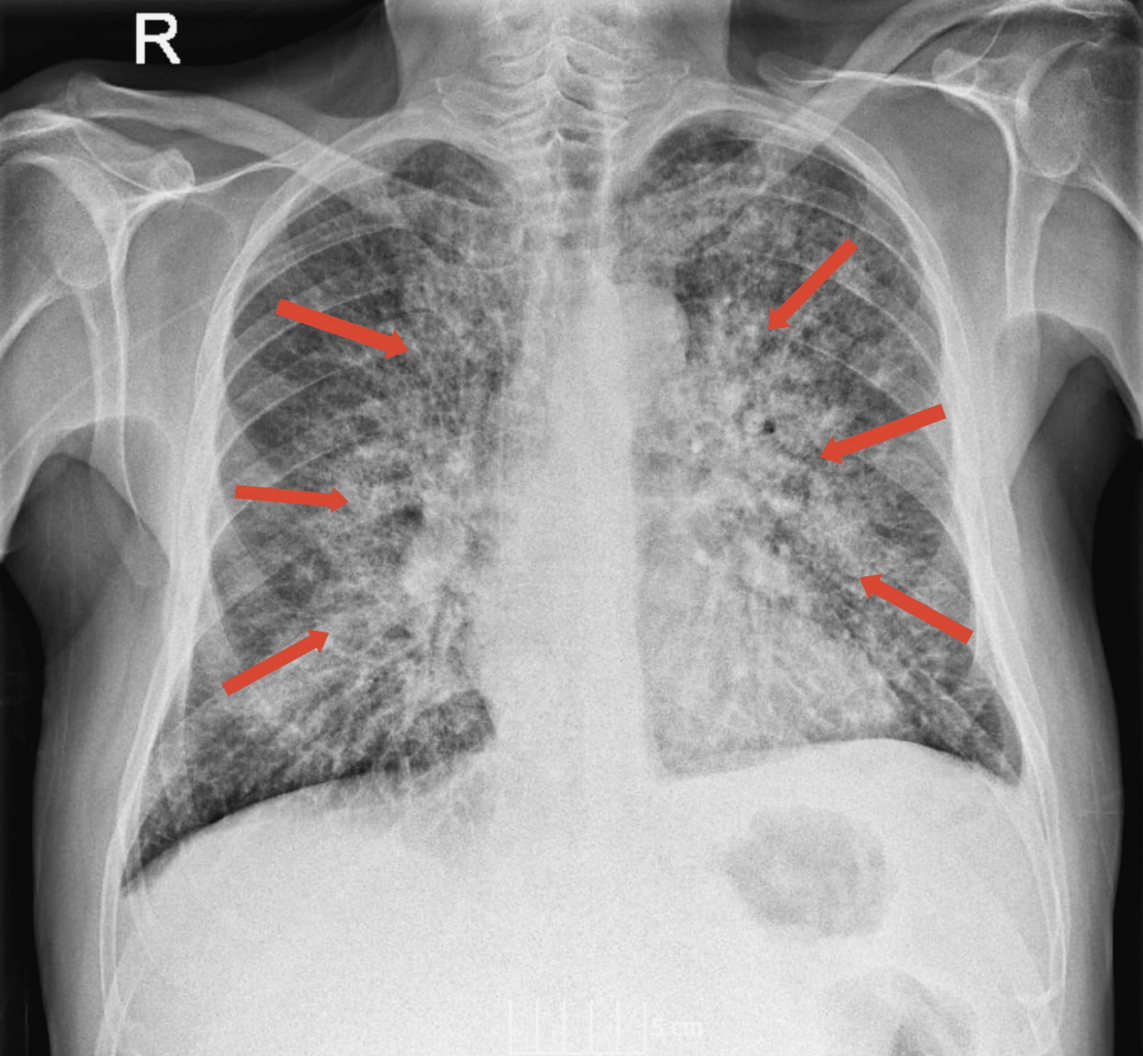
Repeat chest X-ray (48 hours after hospital admission). Repeat chest X-ray (48 hours after hospital admission), showing increase in the prominence of perihilar shadowing in the lungs (indicated by arrows).
